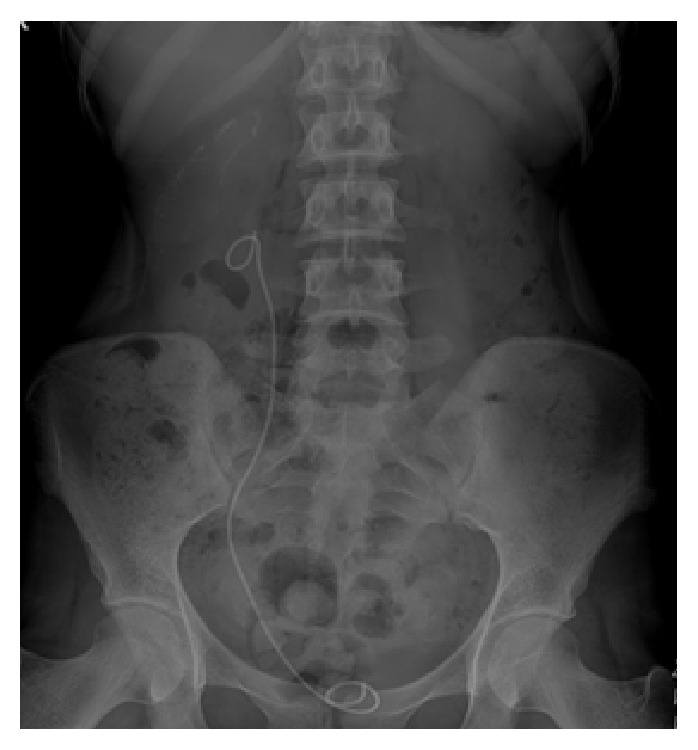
The right kidney was stone-free and perirenal collection of stone fragments was visible on kidney, ureter, and bladder X-ray after the second operation.
